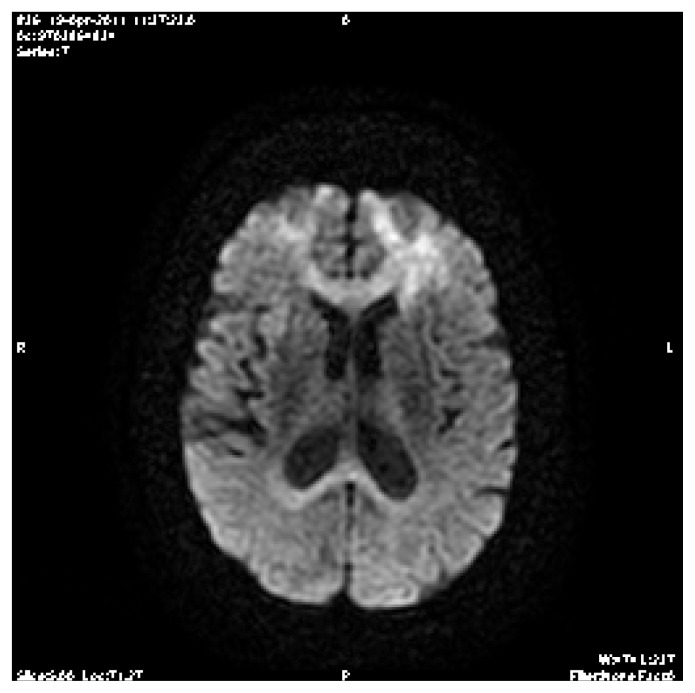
Brain magnetic resonance imaging (MRI) after gadolinium injection showed an image in the axial (FLAIR sequence) showing hyper-intensity lesions in the white matter of the frontal lobes. There is no signal abnormality of the cortex. Note that there is no mass effect on the ventricular cavities or midline structures.
